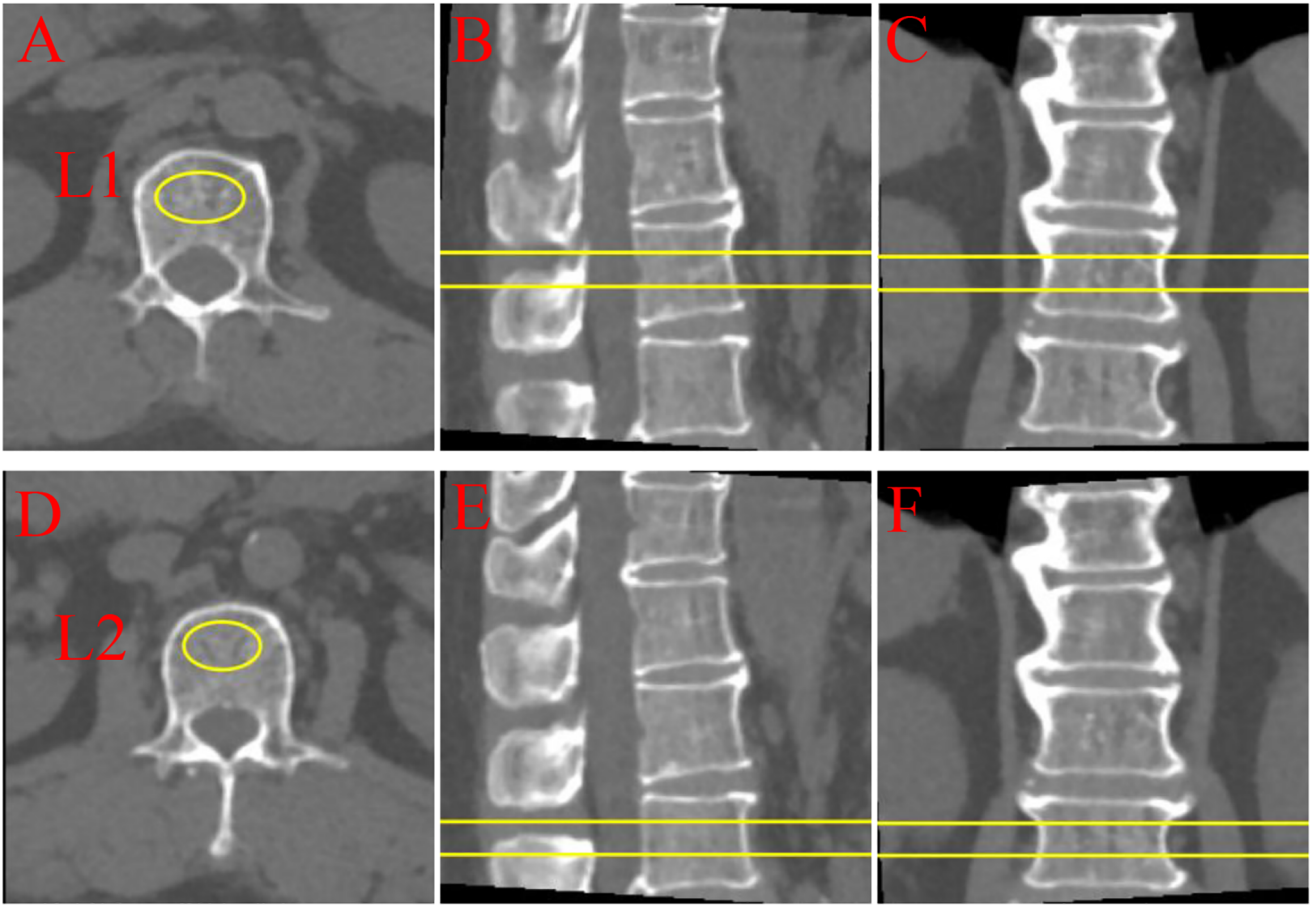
Quantitative computed tomography (QCT) multi-planar imaging of lumbar vertebrae L1 and L2. (A-C) L1 vertebra: (A) Axial, (B) sagittal, and (C) coronal views; (D-F) L2 vertebra: (D) axial, (E) sagittal, and (F) coronal views. Yellow markers and lines indicate regions of interest and measurement planes. QCT provides three-dimensional bone density assessment through orthogonal imaging planes, enabling comprehensive evaluation of vertebral structural characteristics.
